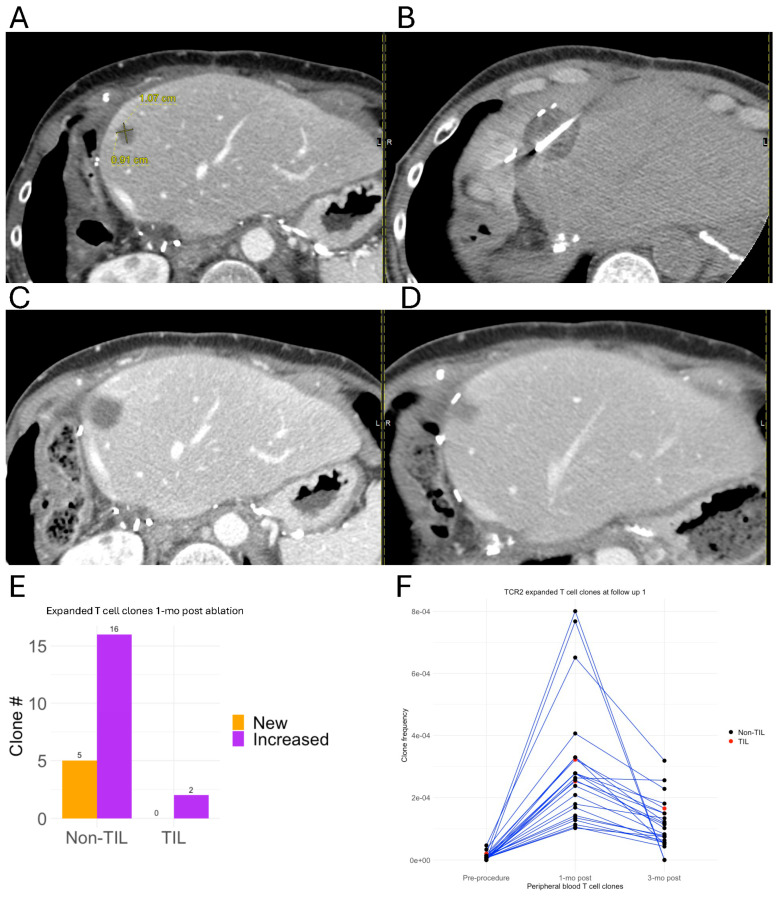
T-cell clonal expansion for a patient with metastatic GIST treated by cryoablation (TCR2). (A) Baseline CT (B) cryoablation (C,D) follow-up CT 1-month and 3-month after cryoablation. (E) Histogram of increased (purple) and new (orange) TILs and non-TILs in the peripheral blood 1 month after procedure. (F) Frequency tracking of T-cell clonotypes expanded 1 month after cryoablation. Each line represents a single T-cell clonotype. TILs and non-TILs represented by red and black spots, respectively.
